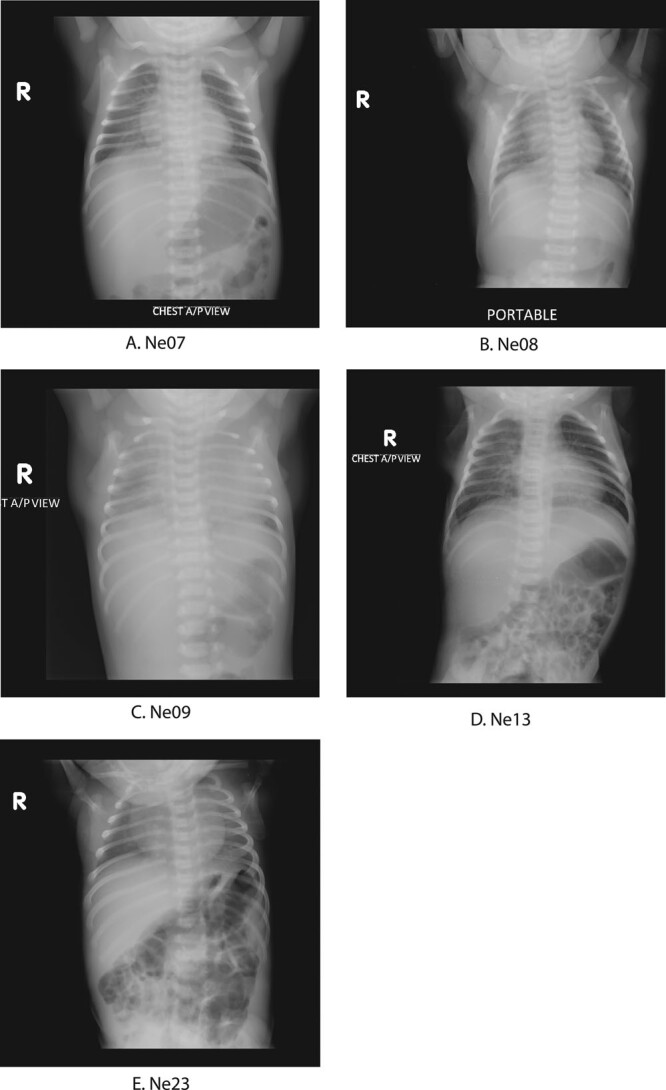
Chest radiograph images of 5 neonates with SARS-CoV-2 infections. A: Chest radiograph of Ne07 showed patchy opacities in right lower lobe suggesting pneumonia. B: Chest radiograph of Ne08 looked normal. C: Chest radiograph of Ne09 showed bilateral ground-glass opacity indicative of SARS-CoV-2. D: Chest radiograph of Ne13 showed few patchy opacities in the right lower perihilar region indicating nonspecific inflammatory lesion in right lower zone. E: Chest radiograph of Ne23 looked normal.
